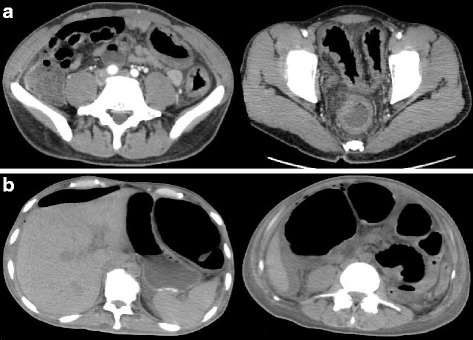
a Contrast-enhanced computed tomography showed long segment marked colonic wall thickening extending from the transverse colon to the distal rectum on the first day of hospitalization. b Computed tomography revealed abdominal free air and dilated loops of colon on the 35th hospital day
